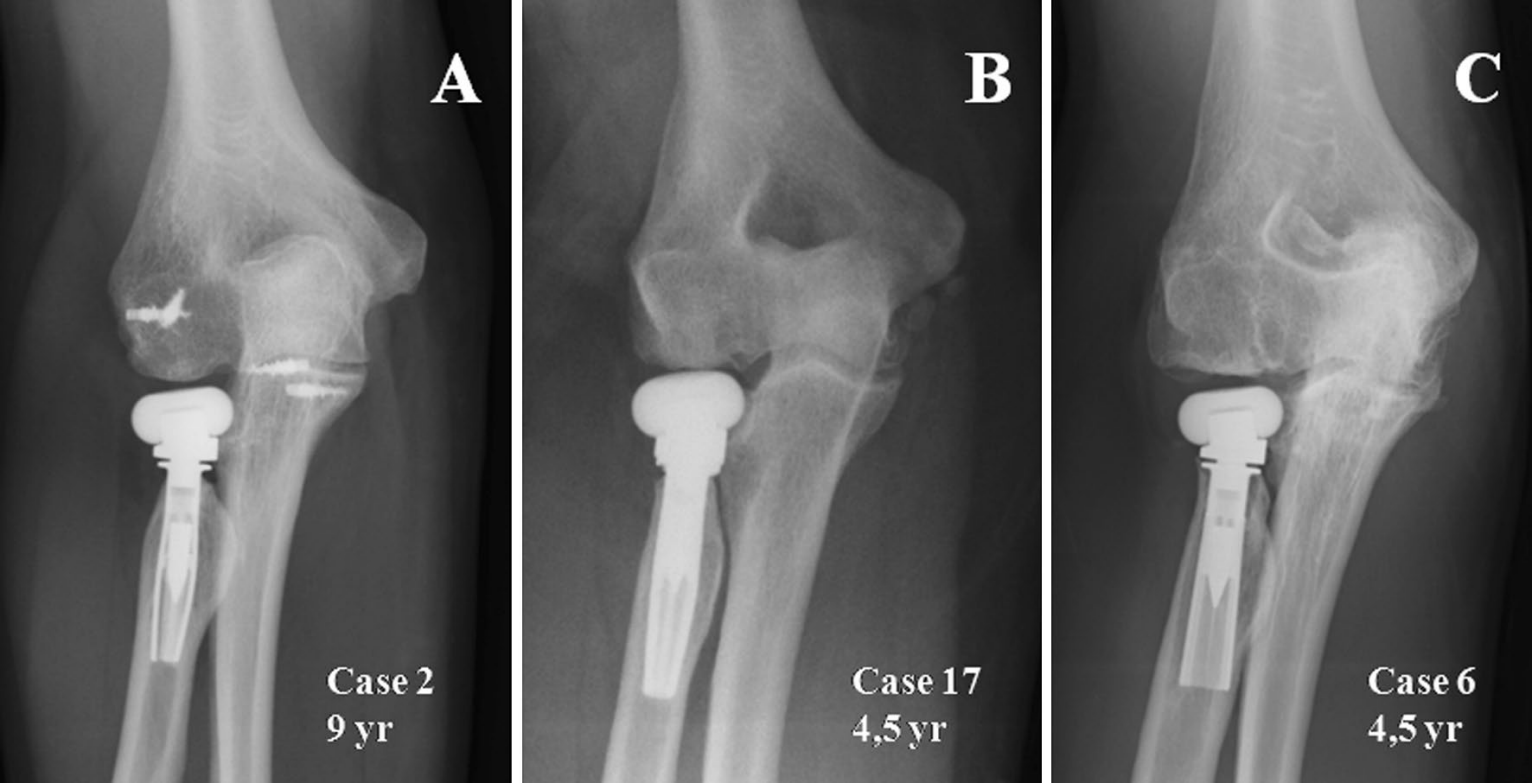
A Case 2, not showing degenerative changes after 106 months, nor changes in the capitellum or medial ulnohumeral osteophyte. B X-ray after 53 months in another patient with type I degenerative changes, according to Brian and Morrey’s classification, with slight changes in the capitellum and no medial osteophyte. C In this case there are severe changes in the capitellum, as well as osteophyte in the medial area of the ulnohumeral joint. We can also see a loss of press fit of the stem, as well as non-progressive radiolucent lines
